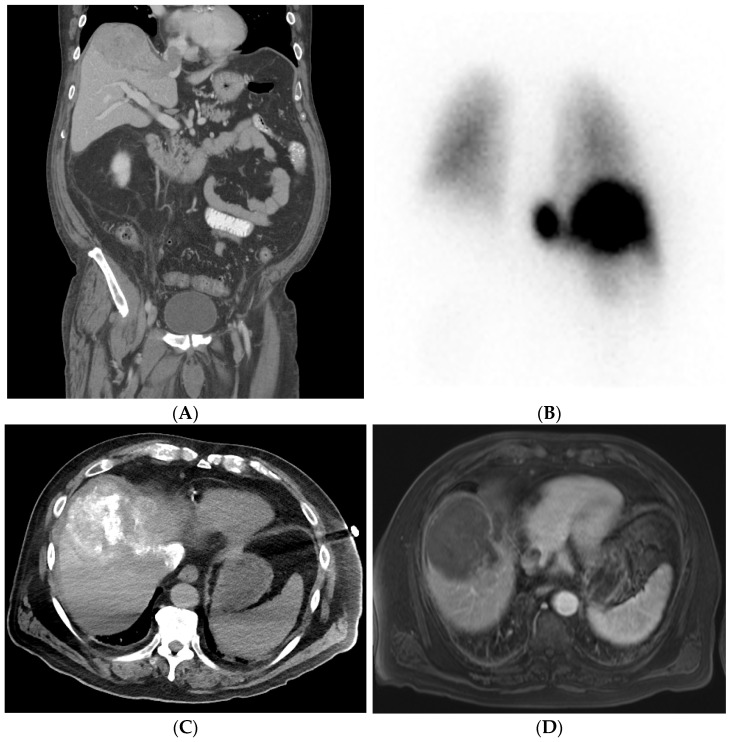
Patient with right lobe HCC with extension into the hepatic vein causing thrombosis. Pre-procedural investigation showed 30% shunt fraction, and therefore the patient was treated with cTACE. (A) Pre-procedural coronal CT shows right lobe HCC lesion with hepatic vein thrombosis. (B) A 99mTc-MAA SPECT/CT shows a lung shunt with a lung shunt fraction of 30%. (C) Intraoperative CT showing good lipiodol uptake in the treated lesion areas. (D) Follow-up MRI showing reduced non-enhancing HCC and hepatic vein thrombus due to good response after treatment.
